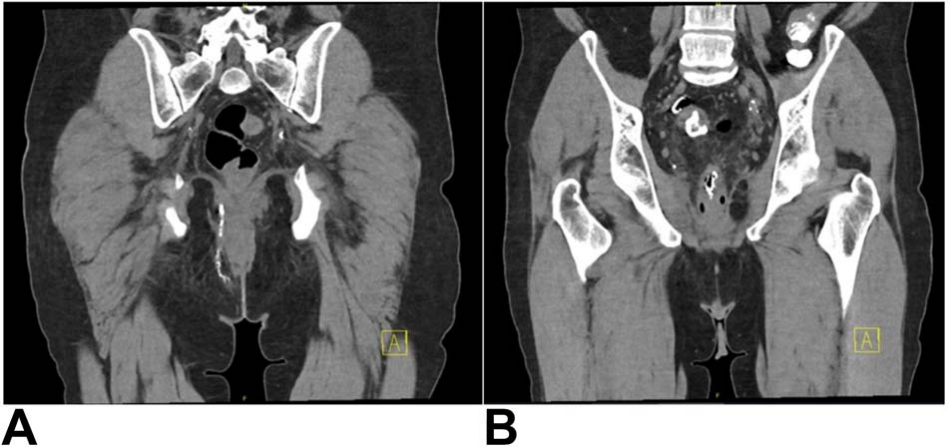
(A) CT fistulogram showing a long fistulous tract extending from the right peri-anal region, superiorly in the ischiorectal fossa, traversing the inferior aspect of the levator ani and extending around the right of the anal canal and rectum; (B) superiorly, it extended into a thick-walled inflamed segment of sigmoid colon with numerous diverticulae; contrast can be seen entering the sigmoidloop.
